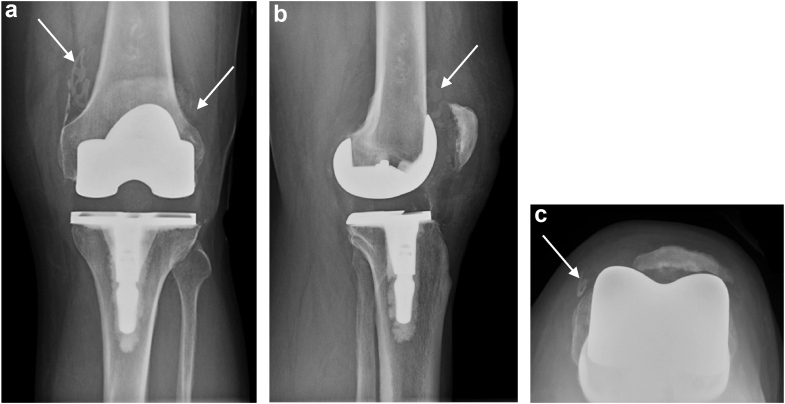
(a-c) Postfailure radiographs at 16 months postoperatively of primary TKA with failed patella implant. Anteroposterior radiograph (a) shows well-fixed implants, soft-tissue swelling, and a knee effusion. Arrows point to displaced polyethylene patella fragments. In lateral view (b), the patellar implant shadow is seen within the supra-patellar pouch (arrow). (c) shows sunrise view with absent polyethylene implant shadow. Note peg cement shadows that suggest the polyethylene pegs are still within the cement. Arrow points to a patella fragment.
